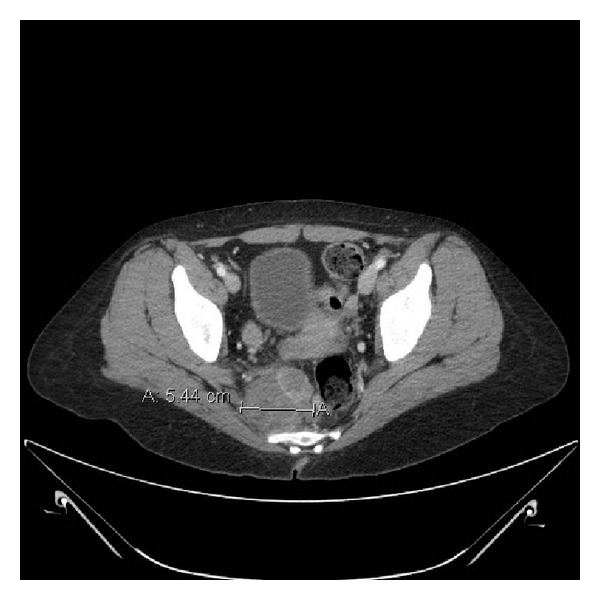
CT scan of the pelvis at the level of the iliac crest reveals a 5.4 cm soft tissue mass. Bones of the pelvis demonstrate abnormal texture.
